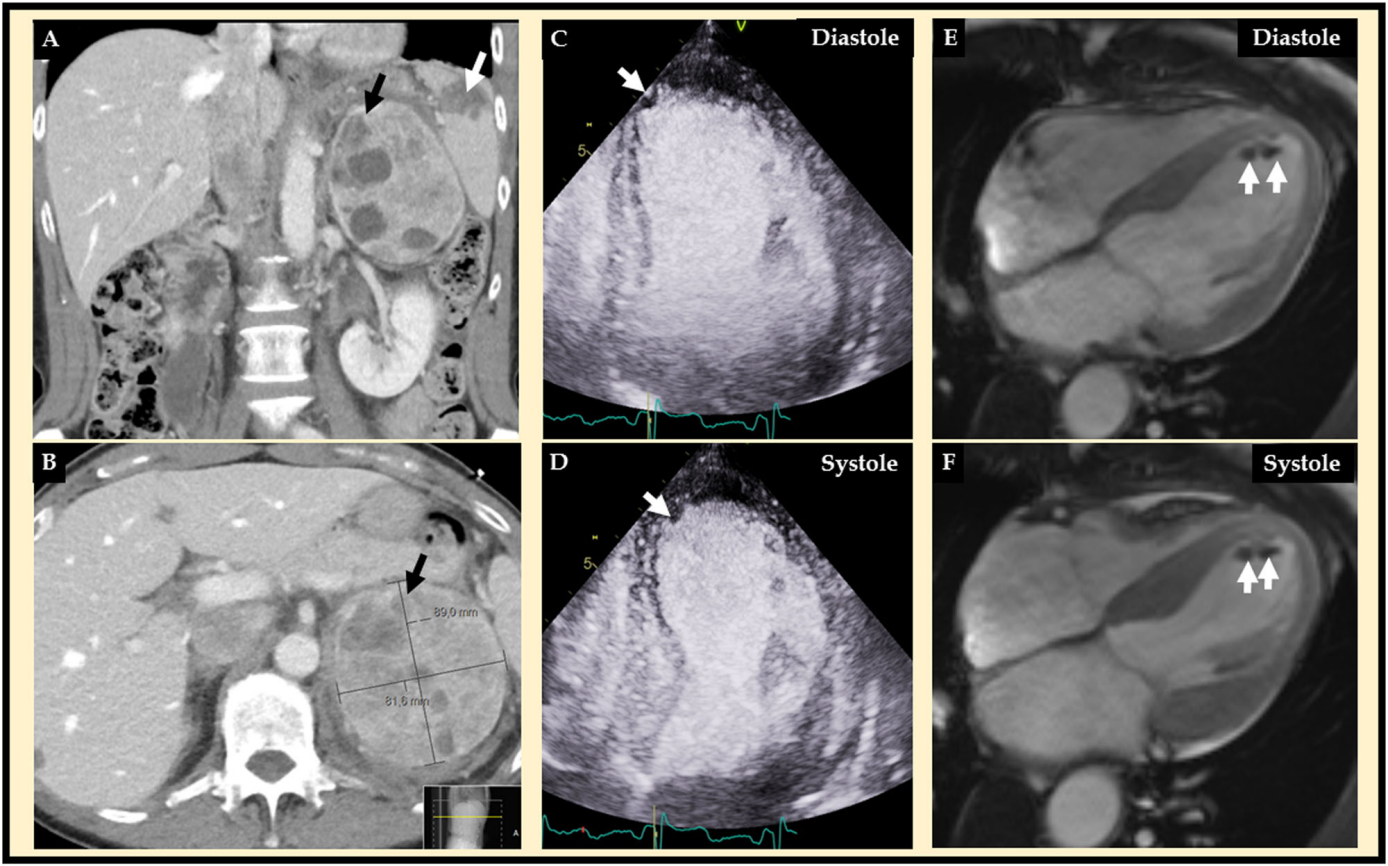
Abdominal computed tomography (CT) (a and b) revealed a left adrenal tumor, which turned out to be a pheochromocytoma (black arrow), and a rupture of spleen (A white arrow) with retroperitoneal bleeding. Contrast echocardiography (c diastole and d systole) revealed mid-apical ballooning typical for takotsubo syndrome with an apical filling defect suggestive of left ventricular thrombus (white arrow). Cardiac magnetic resonance (CMR) imaging (e diastole and f systole) confirmed the mid-apical pattern of takotsubo syndrome and the apical left ventricular thrombus (white arrows)
